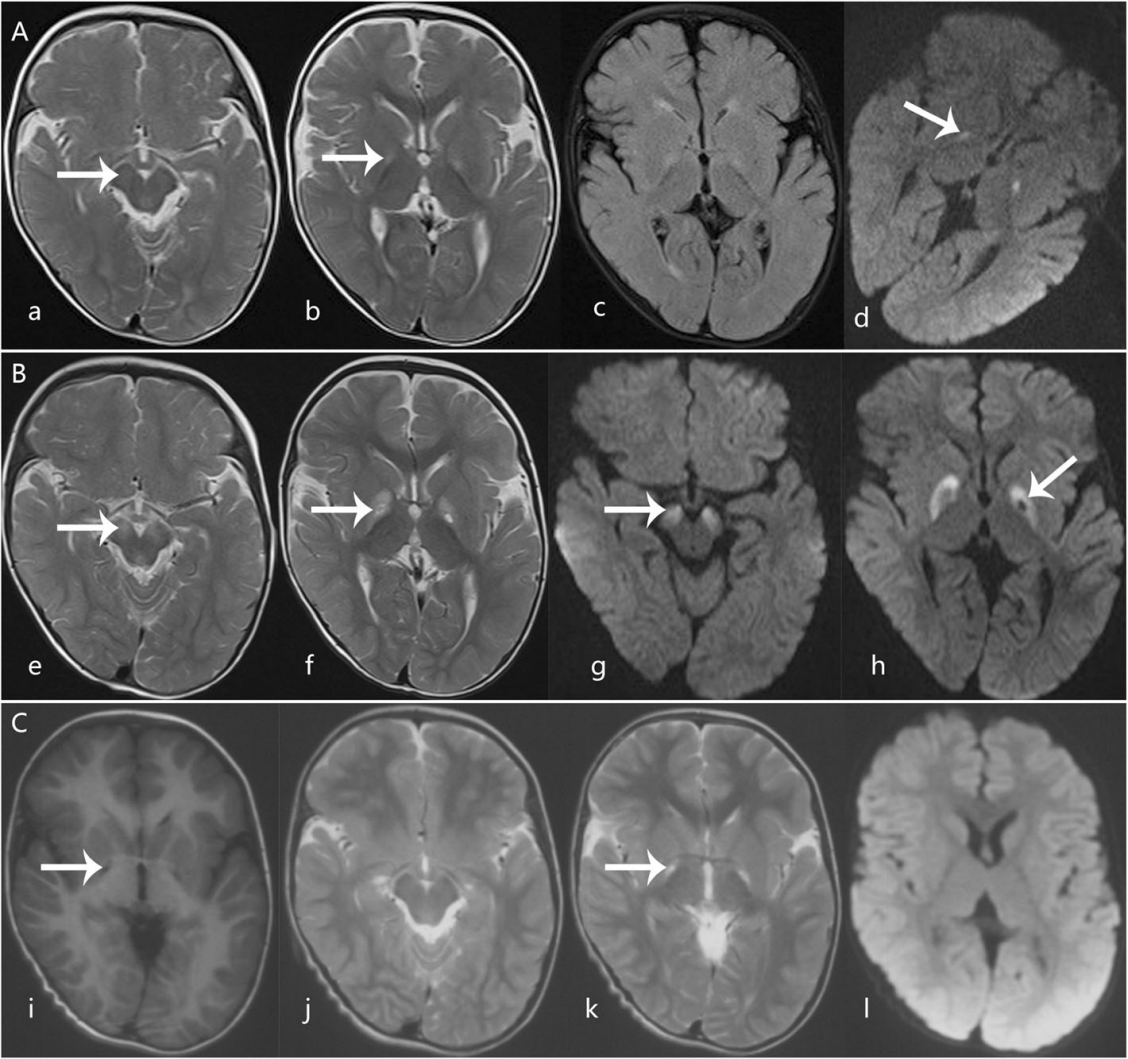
Axial MRI scans from Case 3. T2WI (a, b), FLAIR (c), and diffusion-weighted imaging (DWI) (d) performed at 3 days after disease onset at age 16 months showing high signal in the bilateral globus pallidus and substantia nigra (arrows). e-h, Follow-up images 20 days after clinical onset revealed more conspicuous lesions than the original scan, with a necrotic lesion on the left globus pallidus on T2-weighted (e, f) and diffusion-weighted imaging (g, h)(arrows). i-l, Follow-up images at 2.5 years after onset revealed that lesions on the substantia nigra disappeared (j) and lesions on the globus pallidus were smaller, with well-delineated cysts (i, k) (arrows) and without new lesions and brain atrophy
